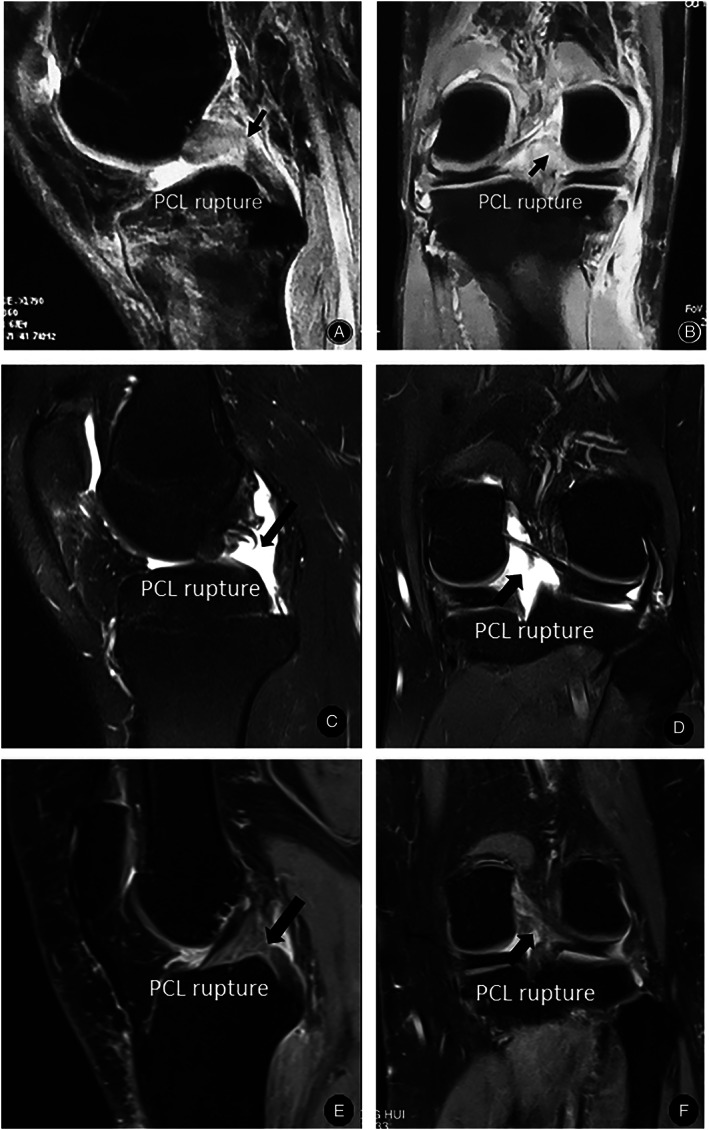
MRI examination of the knee in the reported three cases (A, B for Case 1; C, D for Case 2; E, F for Case 3): posterior cruciate ligament (PCL) rupture can be identified both in sagittal plane (A,C,E) and coronal plane (B,D,F).
